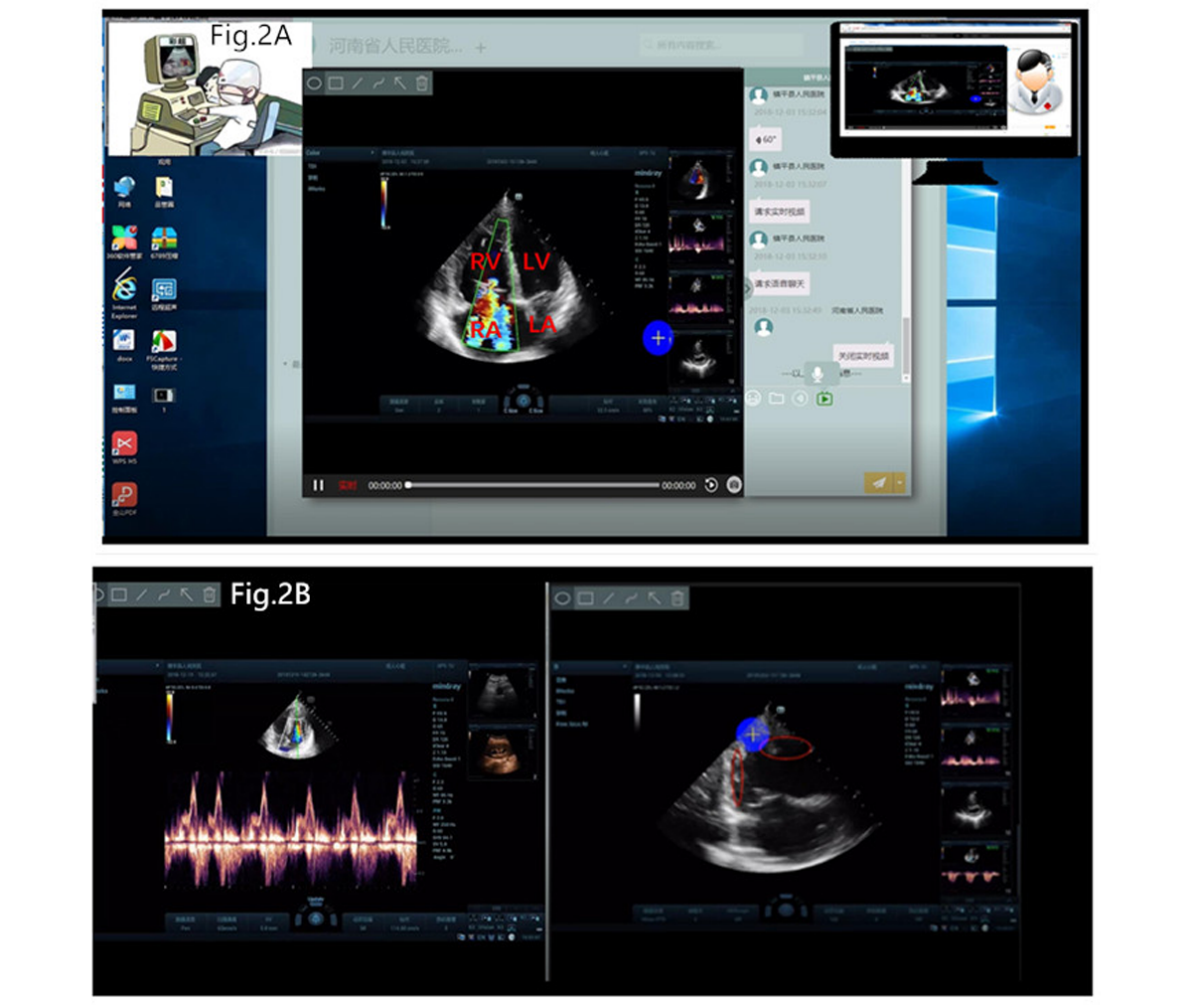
Pictures of remote transthoracic echocardiography consultation. Panel 2A shows the computer interface for remote consultation in one patient. The left upper corner shows an ultrasound physician at the grassroots hospital examining the patient; the right upper corner shows the scene of expert consultation at the superior hospital; in the middle is the image on the 4-chamber view in real time. Panel 2B shows the images of two patients in real time. The left upper corner of the interface is where the electronic marking tool can be found; both parties can add or delete electronic marks at any time, thus achieving three synchronization and double-real-time interaction. The red ellipse in the right insert is the electronic mark. LA: left atrium; LV: left ventricle; RA: right atrium; RV: right ventricle.
